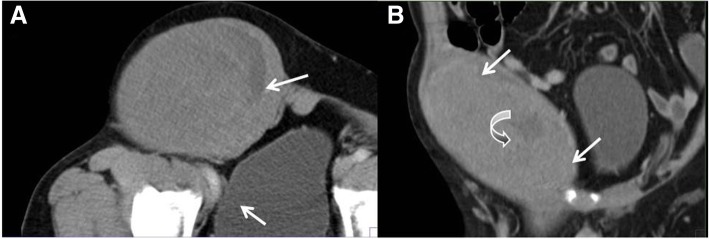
A 51-y-old male patient with an absent scrotum and testis on the right side. a Enhanced CT traverse scanning. b Coronal scanning. Solid masses with a complete capsule (arrow) were visible in the right pelvic cavity. These masses extended into the ipsilateral groin and led to bulging of the abdominal wall. The solid mass was slightly enhanced, and small low-density plaques (dots) were visible in the solid part (curved arrow). Surgery and pathology diagnosed an intra-abdominal undescended testis secondary seminoma
